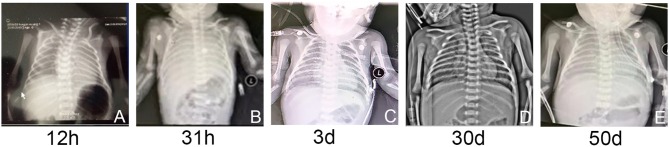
(A–E) The chest radiographs showed varying degrees of decreased transmittance and lung consolidation.
